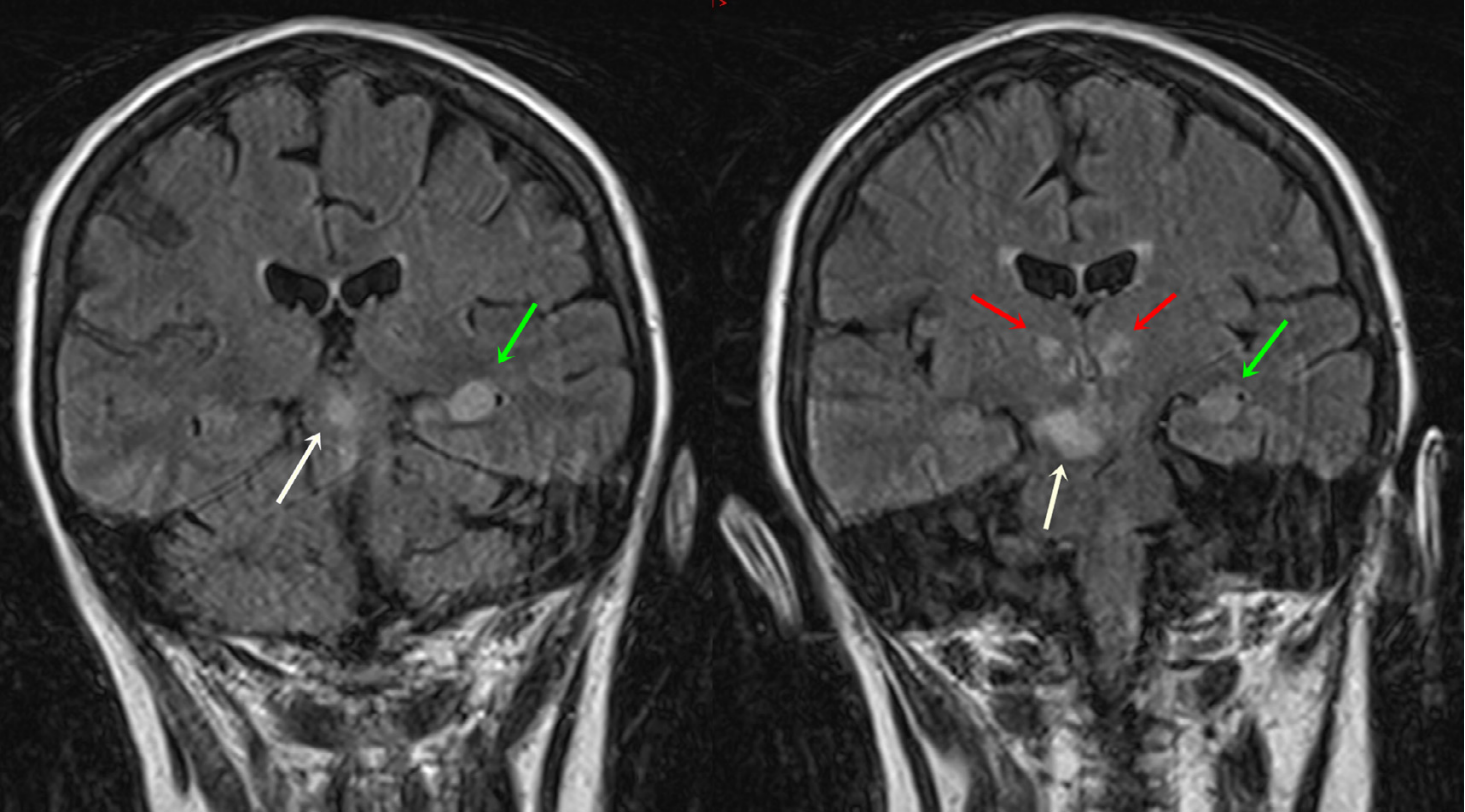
FLAIR images show hyperintense lesion in the central portion of the midbrain (white arrows), bilateral thalami (red arrows), and left hippocampus (green arrows).
